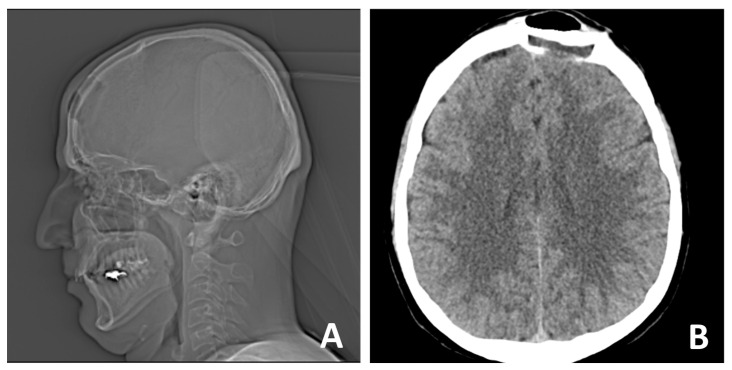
Control (A) X-ray and (B) CT was performed on the following day.
